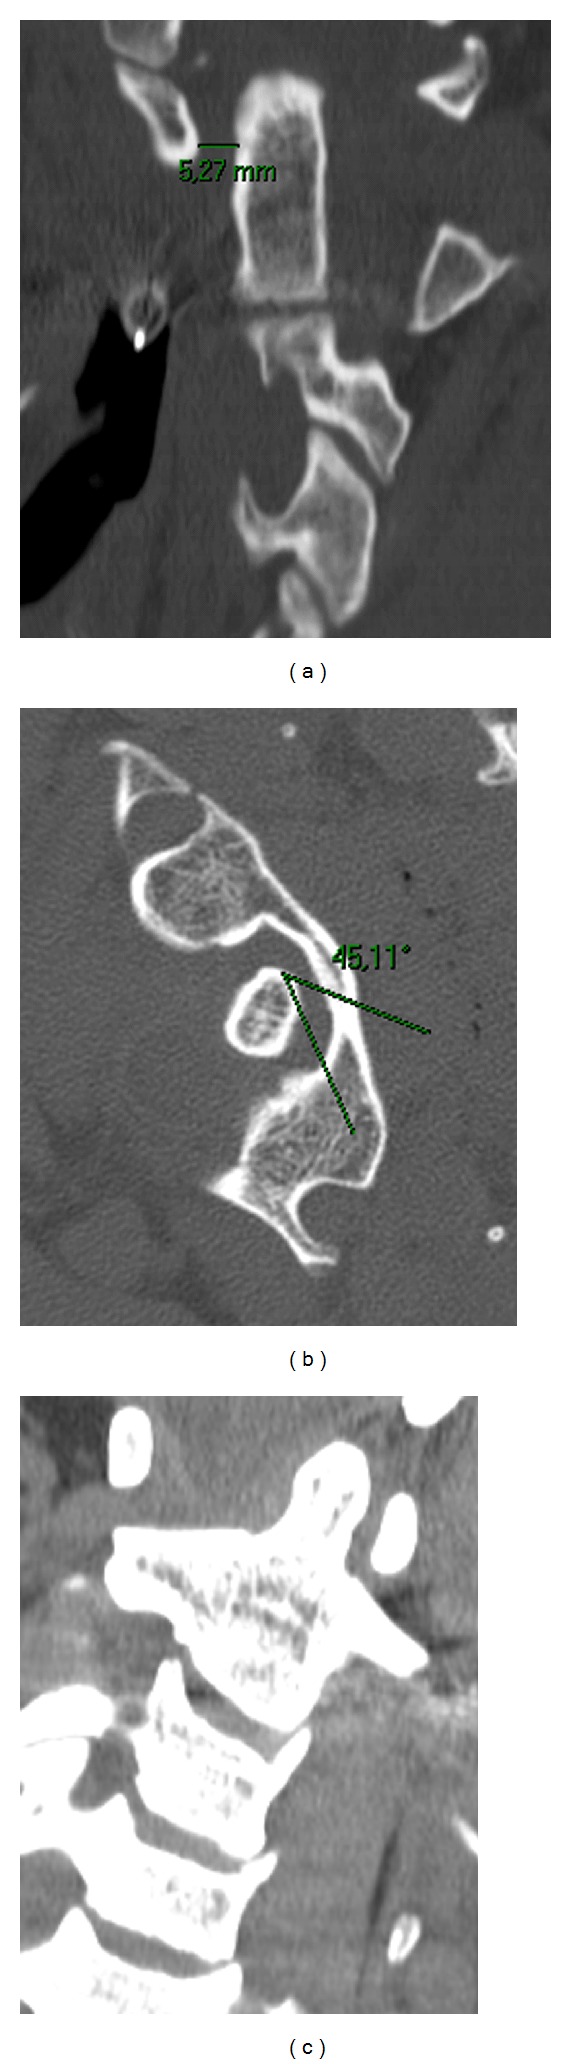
Computed tomography scans showing an atlanto-dental interval greater than 5 mm (a), 45 degrees of rotation (b), and asymmetry of the lateral masses of C1 (c).
